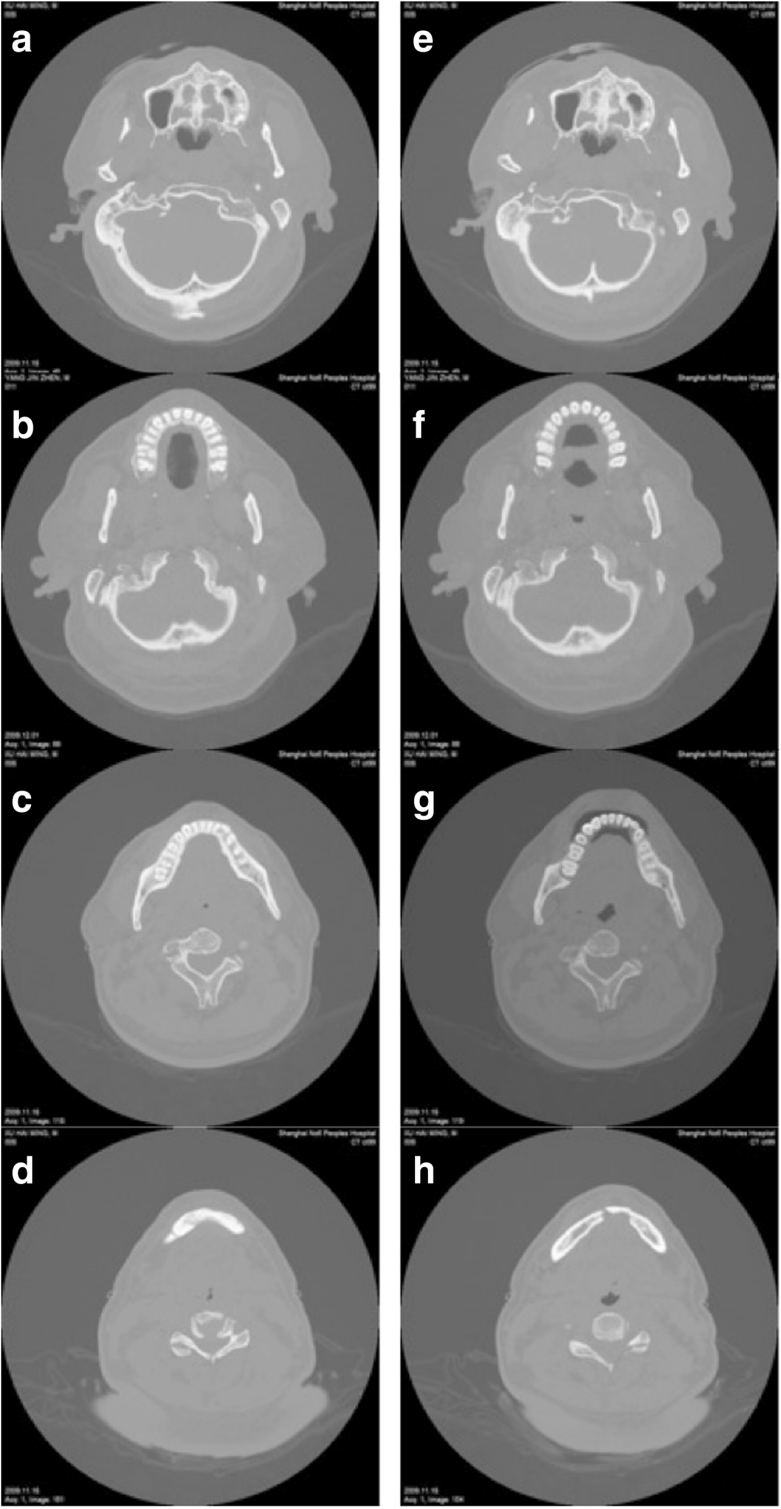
CT scan of responders under the condition of sleep apnea. Without OA in place: nasopharynx (a), velopharynx (b), glossopharynx (c), epiglotopharynx (d). With OA in place: nasopharynx (e), velopharynx (f), glossopharynx (g), epiglotopharynx (h)
